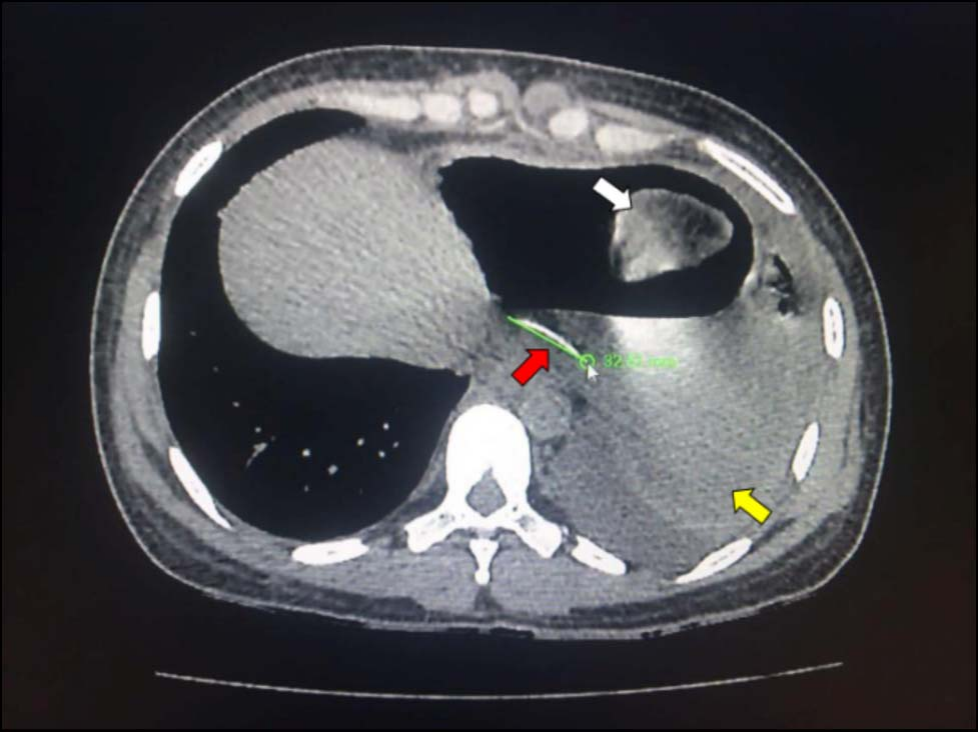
Chest CT showing fishbone measuring 3.4 cm (red arrow), pulmonary consolidation (white arrow), and pleural effusion (yellow arrow) on the left. CT, computed tomography.
